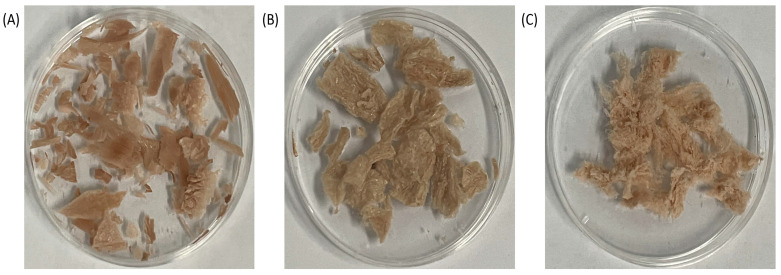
Morphologies of the polysaccharide from *Sinopodophyllum hexandrum* fruit (SHP) and ultrasonic–treated (250 W and 500 W) polysaccharides (SHP1 and SHP2). (A) Morphology of SHP. (B) Morphology of SHP1. (C) Morphology of SHP2.
